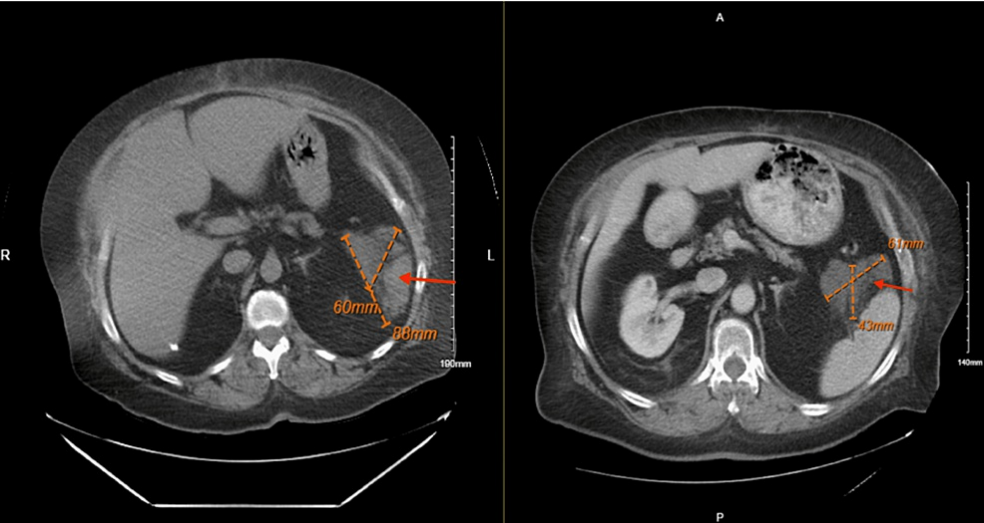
Left image: CT from a year prior with pseudocyst (red arrow) measuring 8.8 cm x 6.0 cm or 88 mm x 60 mm. Right image: most recent CT with pseudocyst (red arrow) measuring 6.1 cm x 4.3 cm or 61 mm x 43 mm cm: centimeters; mm: millimeters
